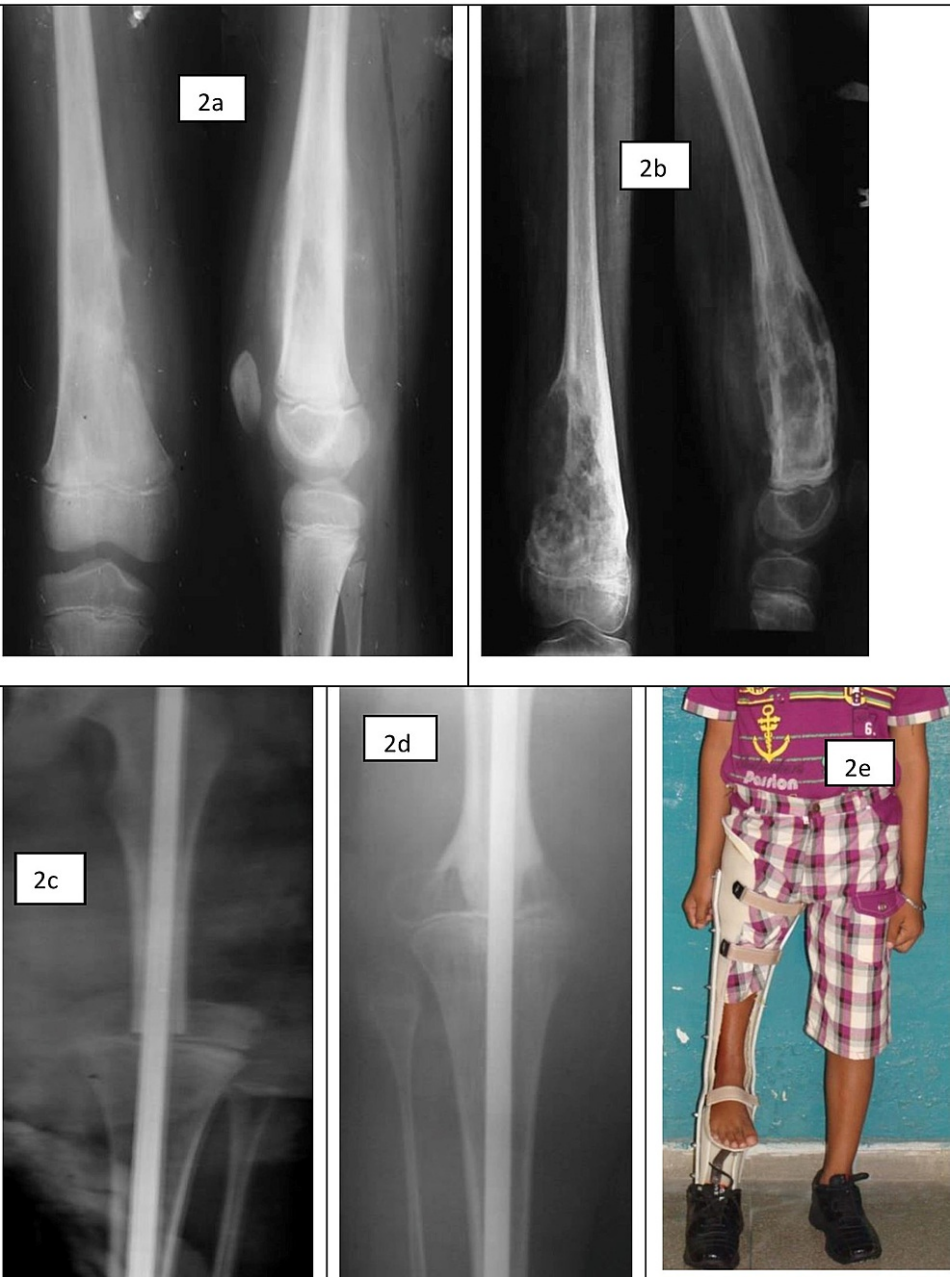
A 12-year-old boy with a diagnosis of osteosarcoma of the distal thigh (First row) showing radiographs (anteroposterior and lateral views) of the femur with an osteolytic lesion, pre-chemotherapy (2a) and post-chemotherapy films (2b), (second row) radiographs showing postoperative osteosynthesis of the partially salvaged limb with intramedullary nailing, immediate postop with adequate alignment (2c); and one-year follow-up with callus formation/healed bone ends (2d), clinical picture with the custom-made extension prosthesis in functional position (2e).
